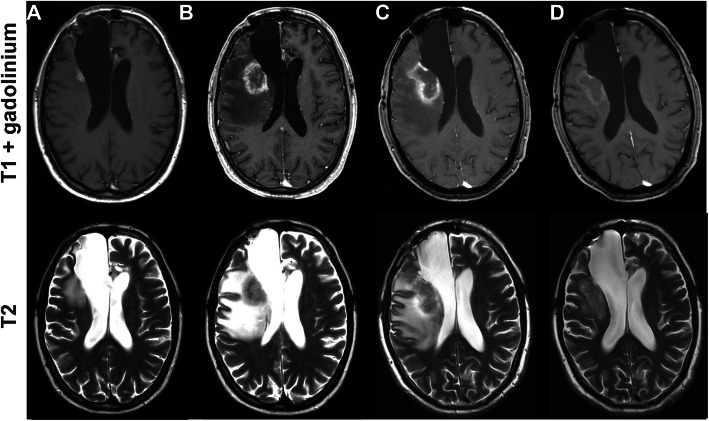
MRI scans of a 34 year old patient with IDH-mutated astrocytoma. a MRI revealed a small recurrent tumor adjacent to the dorsal resection cavity with small surrounding edema. b The patient was treated with re-radiation therapy with 35 Gy and concomitant temozolomide. First MRI after the treatment showed an increase of contrast enhancement and edema which was diagnosed as radiation necrosis. c Therapy with 8 mg of dexamethasone did neither improve the MRI nor the clinical symptoms and bevacizumab 7.5 mg/kg was administered as a single-shot. d First scan one month later displayed a marked reduction in contrast enhancement and of the edema. Treatment with dexamethasone could be stopped. The follow up 3 months later was stable (not shown)
